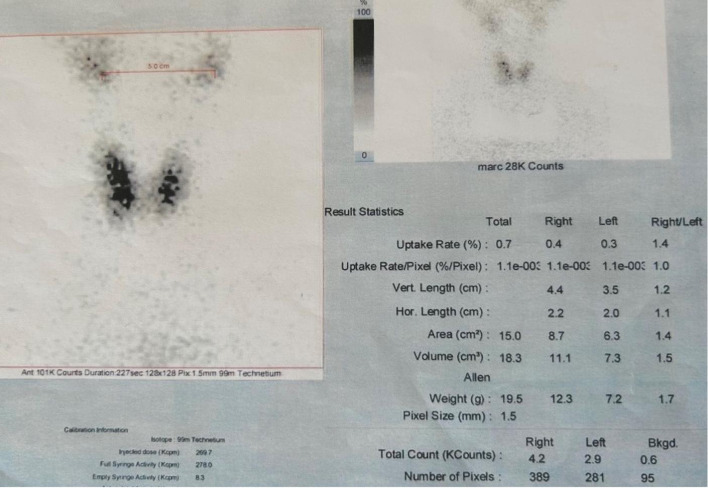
Thyroid scintigraphy using 99m technetium pertechnetate (thyroid scan) approximately 30 days after the initial biochemical diagnosis. Normal uptake of the marker was seen in both lobes of the thyroid gland.
